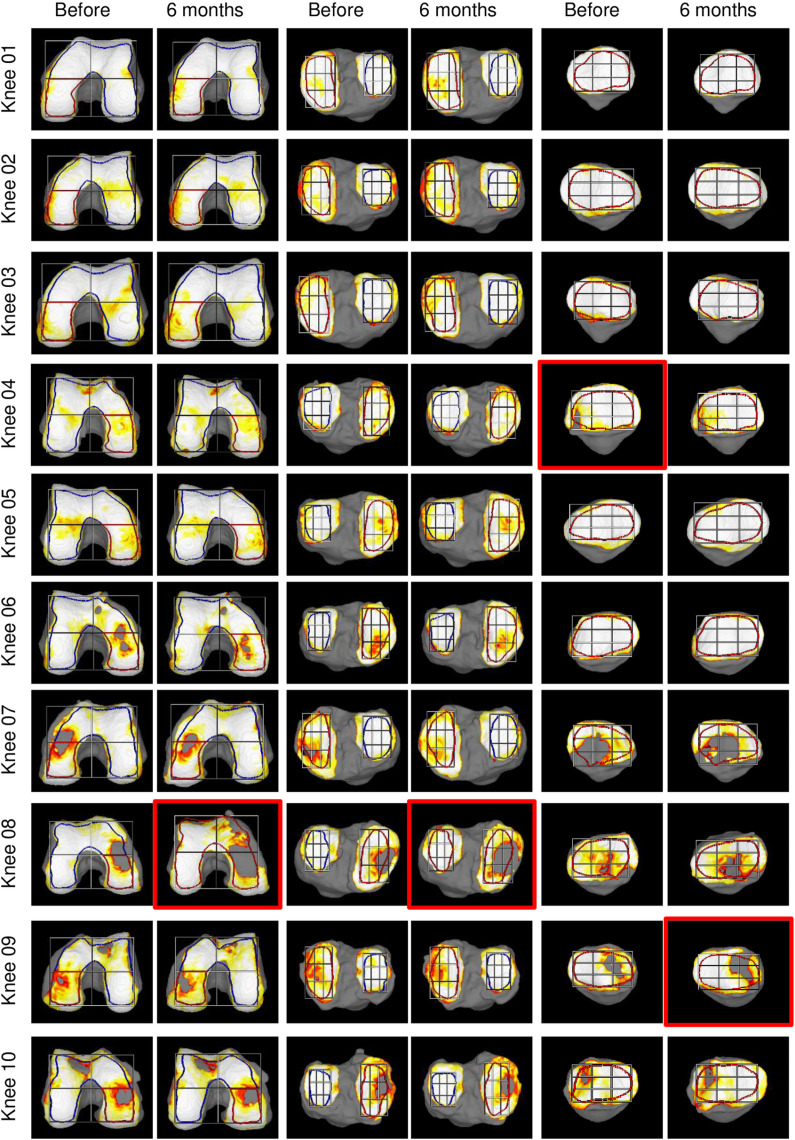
Reconstructed 3D MRI for knee cartilage and ROIs in Knee 01-10, representing the first half of the complete series. The 21 knees are arranged in descending order of cartilage thickness in the PMF region. The red frame indicates cases in which the automatic ROI setting was inappropriate, so another ROI was copied. The blue frame indicates cases in which the automatic ROI settings for two areas were inappropriate, so one was set manually and the other was copied from it.
